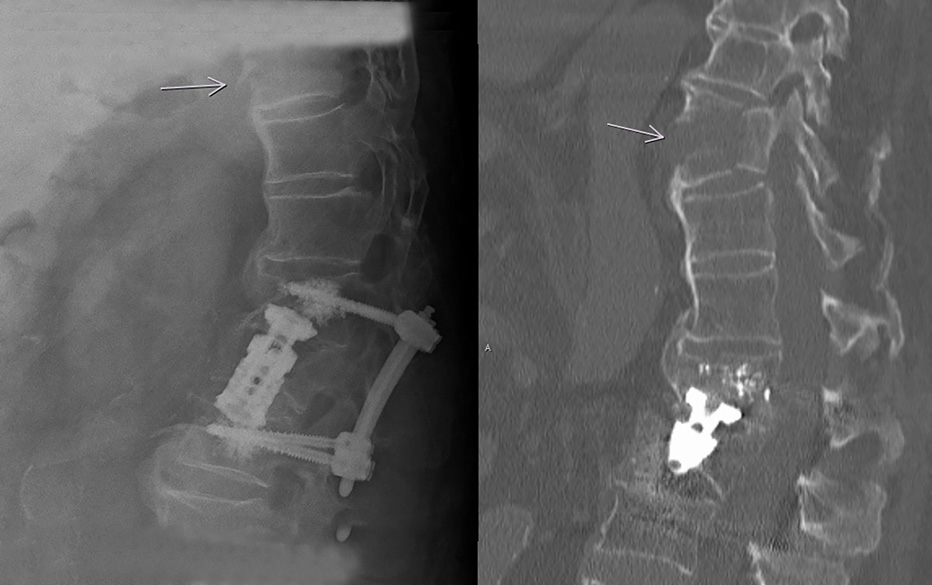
Fracture in an 87-year-old man presenting with immobilizing lumbar pain after a fall. Radiography (on the left) shows spondylodesis of a L2- fracture. At the border of the x-ray a step in the ventral cortex of TH 10 and a subtle fracture line of the vertebral body can be found. The performed CT-scan (on the right) shows the unstable distraction fracture
